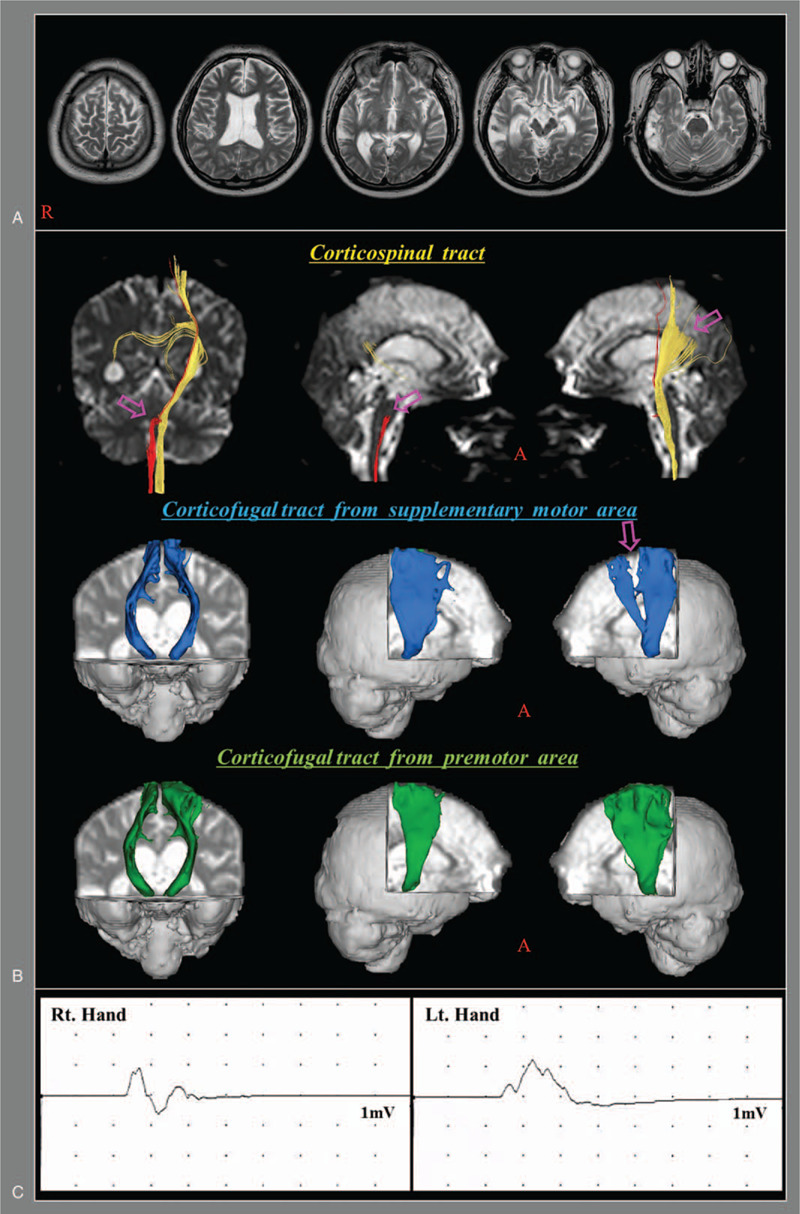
A. T2-weighted brain magnetic resonance images obtained at 32 months after onset show a leukomalactic lesion in the right temporal lobe. B. On 32-month diffusion tensor tractography, the right corticospinal tract has a discontinuation at the pontine level (arrow), whereas the left corticospinal tract shows partial tearing at the subcortical white matter (arrow). The corticofugal tract from the left supplementary motor area shows partial tearing at the subcortical white matter (arrow). C. Motor evoked potentials obtained from abductor pollicis brevis (APB) and tibialis anterior (TA) muscles (right side APB latency: 22.3 msec, amplitude: 1.6 mV; left side APB latency: 22.8 msec, amplitude: 1.5 mV).
